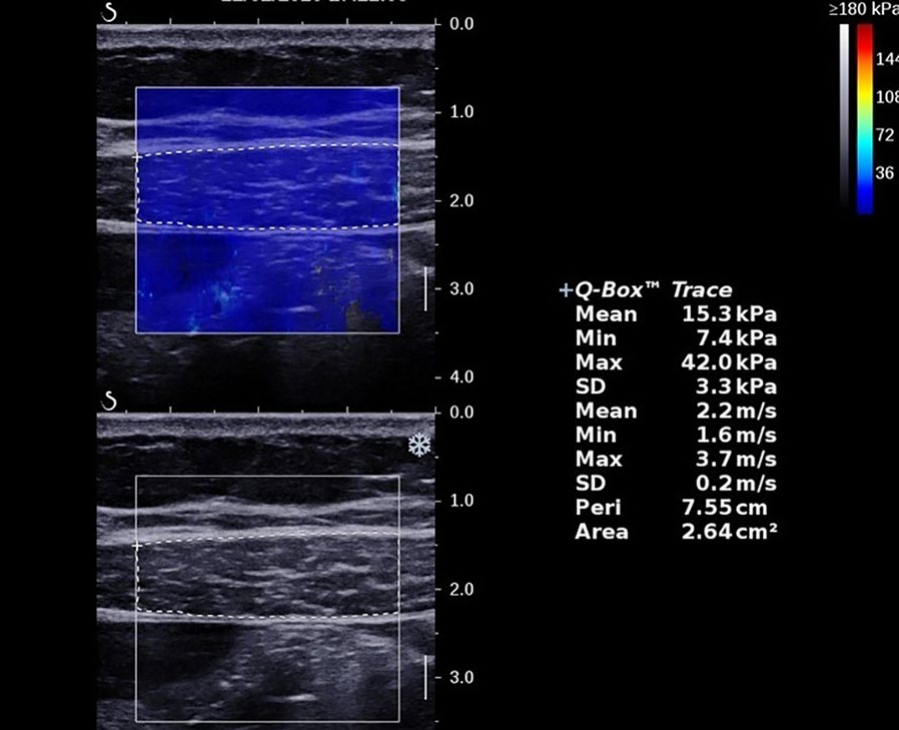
Ultrasound images of shear wave speed measurement in upper right rectus abdominis. A new region of interest (dotted line) was drawn to obtain the parameter of mean shear wave speed
